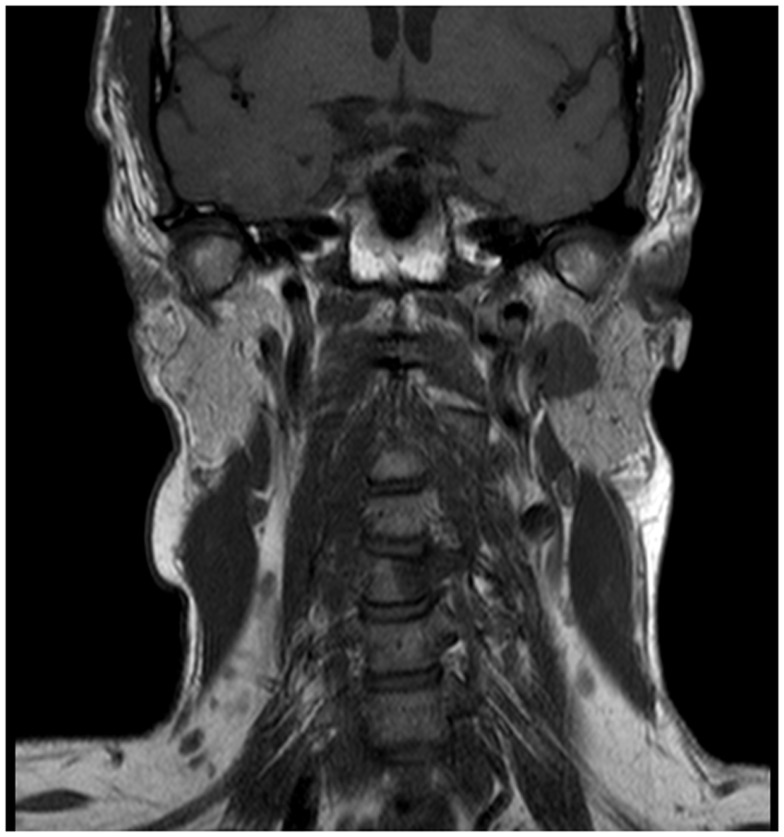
Magnetic resonance imaging showing the left parotid gland mass in the coronal plane.
